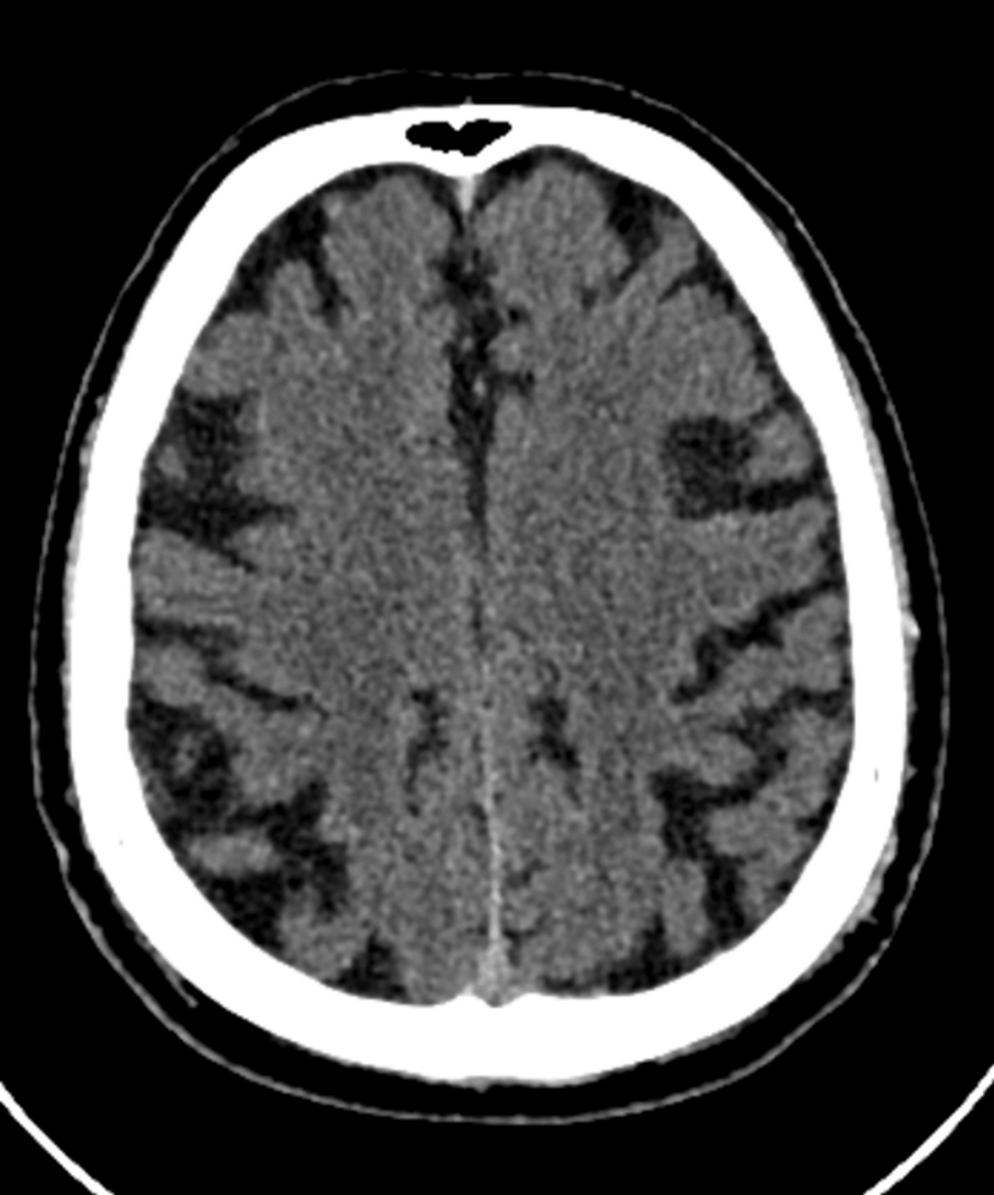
Axial CT scan image of the brain showing mild cortical atrophy
